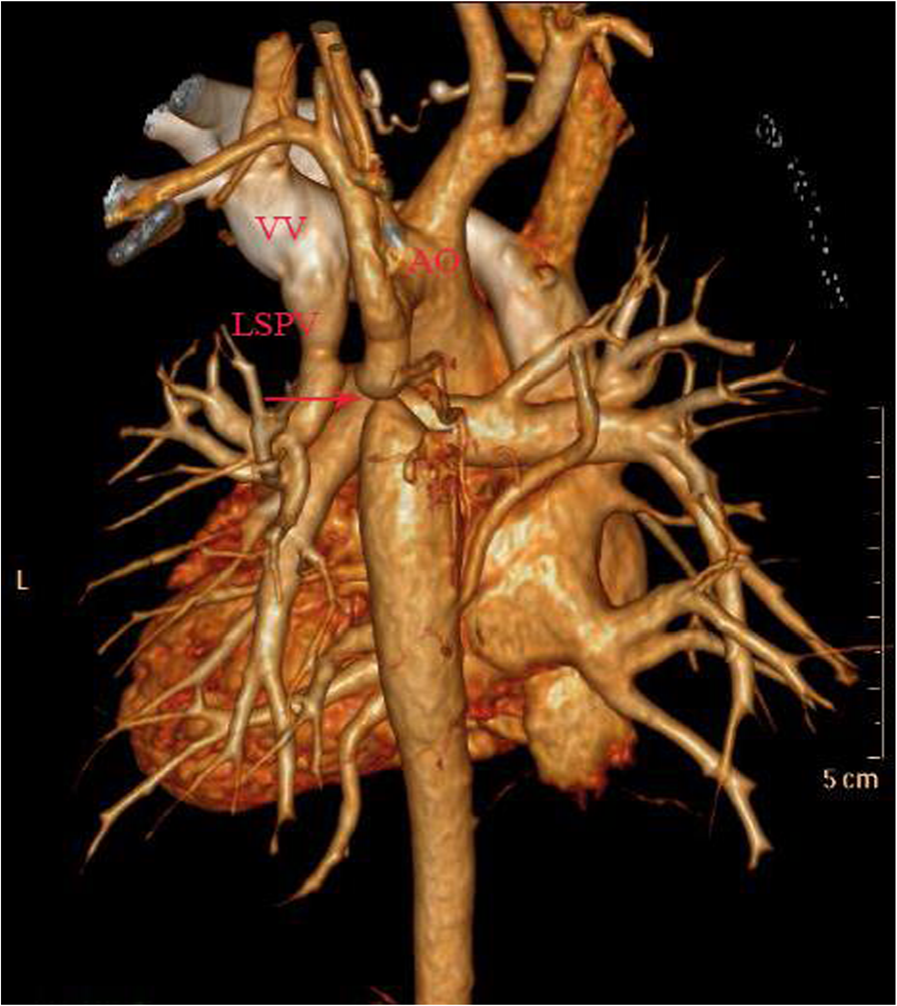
Preoperative computed tomography demonstrating the right superior pulmonary vein draining into the vertical vein and the coarctation of the aortic arch (AO = aorta; VV = vertical vein; LSPV = left superior pulmonary vein; *red arrow* = coarctation of the arch)
